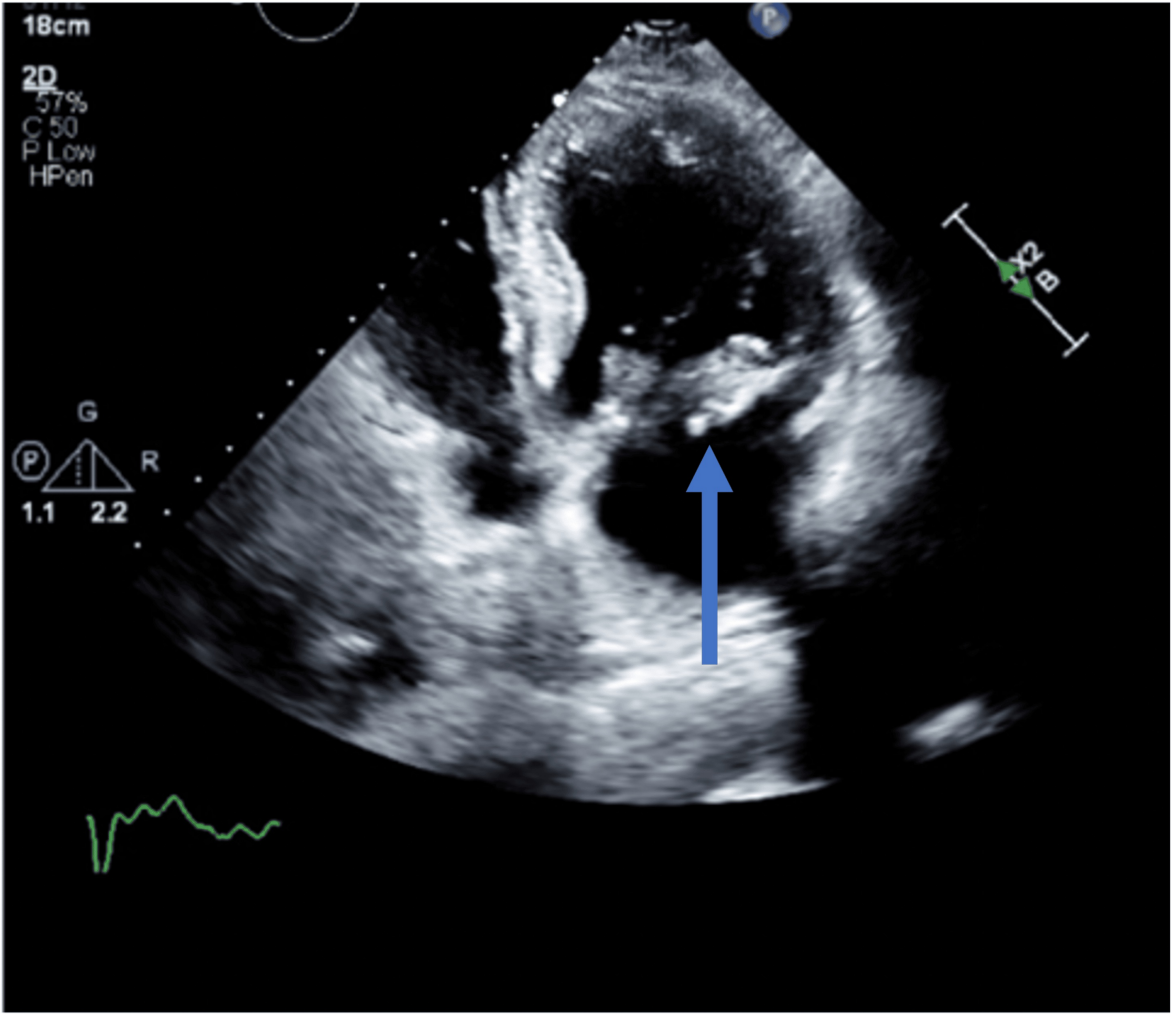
Echocardiogram of mitral valve vegetation
